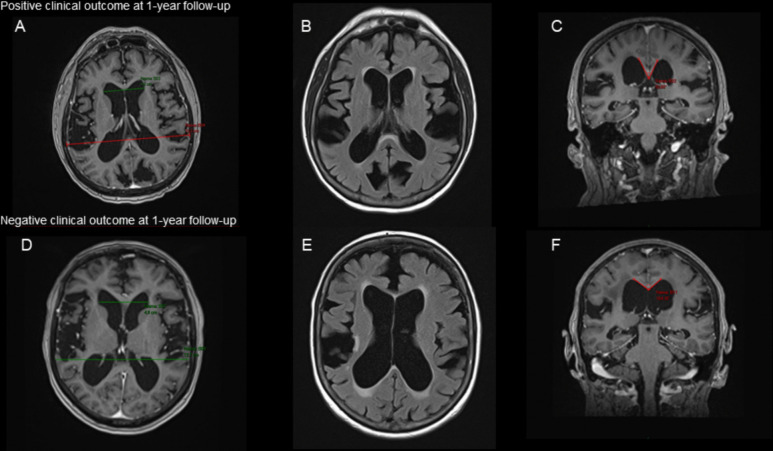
Brain MRI of two patients who underwent shunt placement, performed during pre-shunt clinical assessment. A–C Images from a patient with a positive clinical outcome at 1-year follow-up. A Axial T1-weighted image shows an Evans Index of 0.3409. B Axial FLAIR image shows mild white matter hyperintensities (Fazekas score 0). C Coronal T1-weighted image at the posterior commissure demonstrates a callosal angle of 52.65° and DESH pattern. D–F Images from a patient with a negative outcome at 1-year. D Axial T1-weighted image shows an Evans Index of 0.3701. E Axial FLAIR image shows more pronounced asymmetric white matter disease hyperintensities. F Coronal T1-weighted image at the posterior commissure demonstrates a callosal angle of 107.49°, DESH pattern was absent
